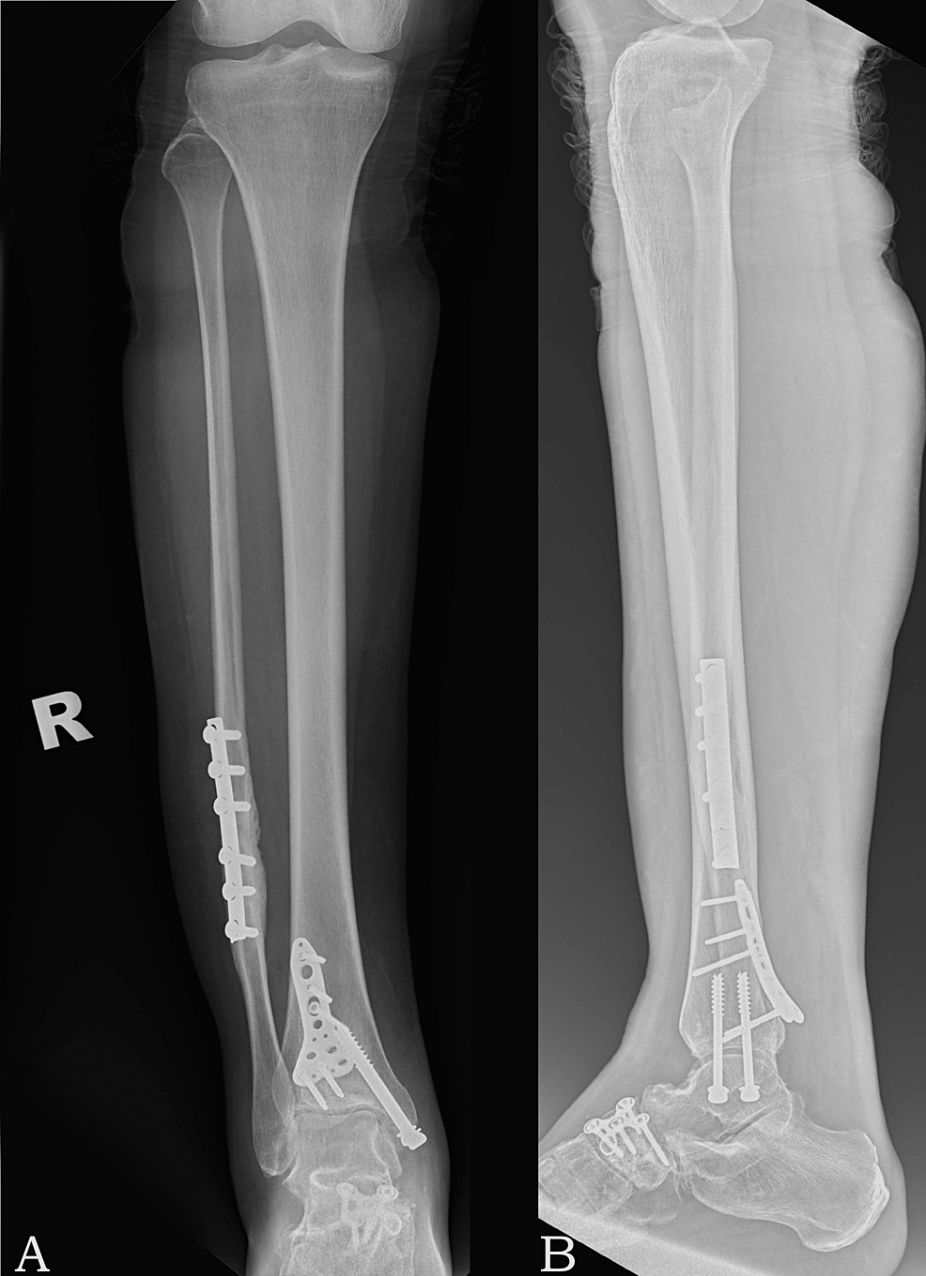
Postoperative tibia and fibula anteroposterior (AP) and lateral radiographs with implants in situ. (A) AP view of the right tibia fibula radiograph and (B) lateral view of the right tibia fibula radiograph.
